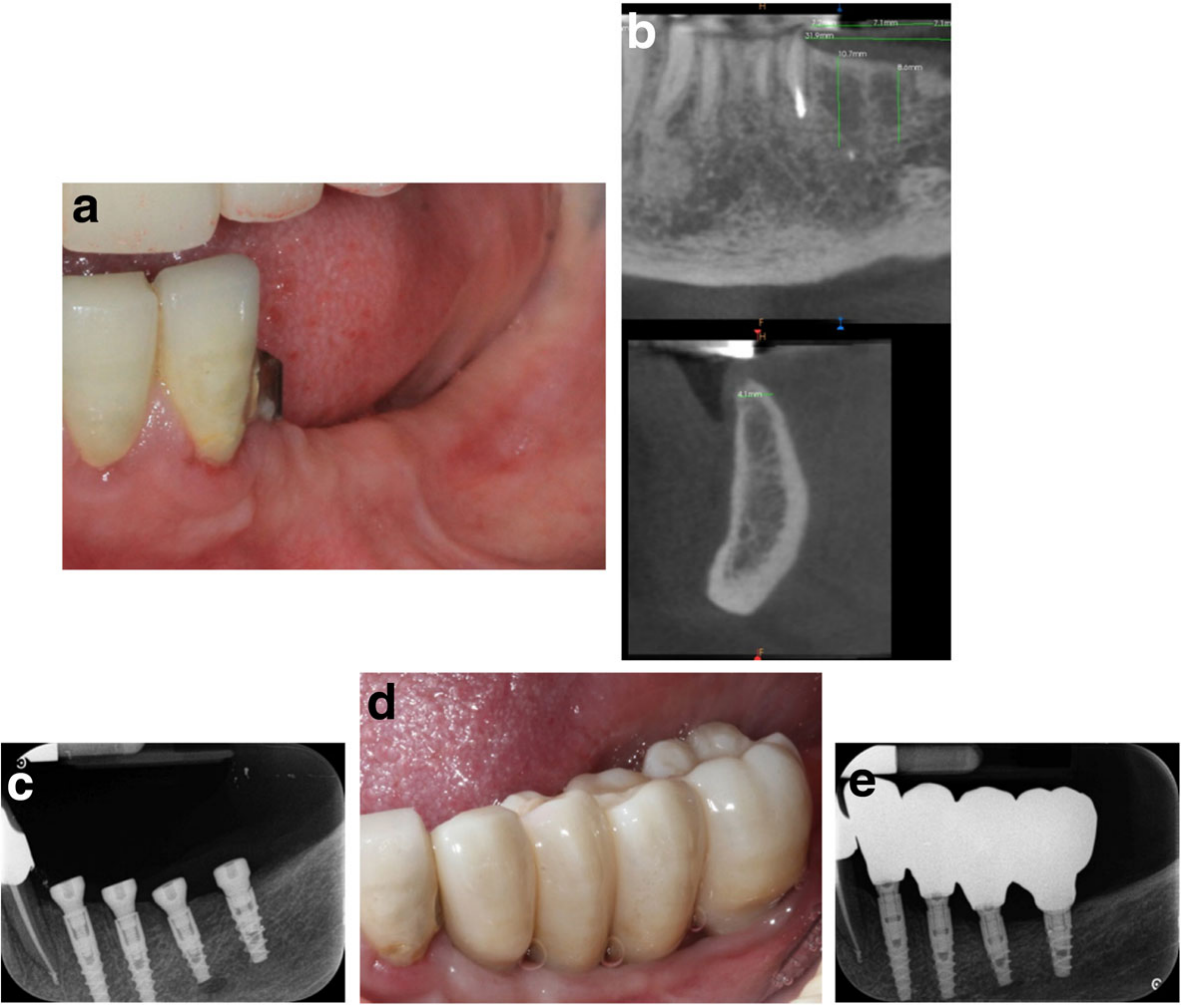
Example of another case involved in the study. a Preoperative view –premolars and molars are missing in left mandible. b Preoperative CT scan. The width of the ridge was around 4 mm. c Baseline periapical radiograph. Four narrow diameter implants were placed to restore the area. d Buccal view of the final full-contour zirconia restoration. e Periapical radiograph at 1 year after loading
